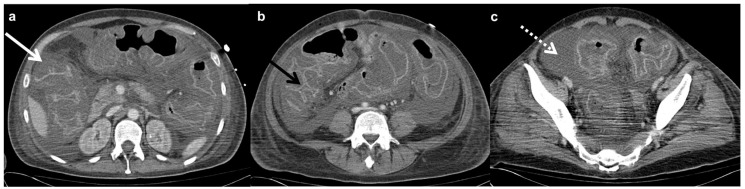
CT imaging used to identify *C. diff.* with an axial CT scan demonstrates low-attenuation wall thickening involving the entire length of the colon with intense mucosal enhancement (white arrow, (a)); the enhancing mucosa is stretched over the edematous haustral folds, resembling an accordion even in the absence of intra-luminal contrast (black arrow, (b)). A large amount of ascites is also present (dashed arrow, (c)) [59].
